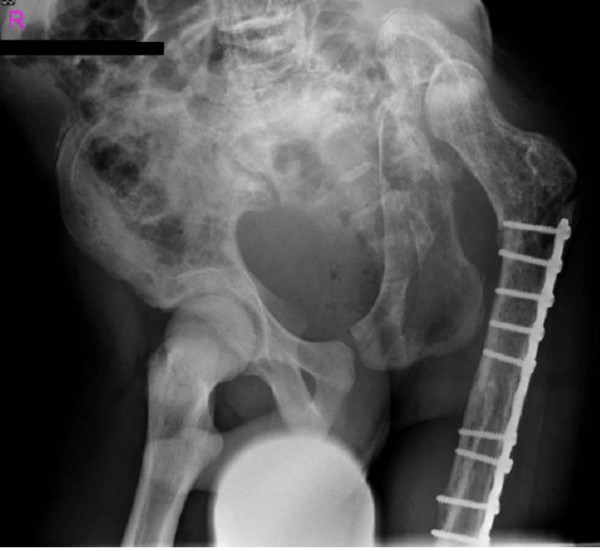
(Patient I). Anteroposterior pelvis radiograph showed complete dissolution of the left hemipelvis, with superior migration of the femoral head. At this stage we fixed the fracture with a long Gamma nail.
